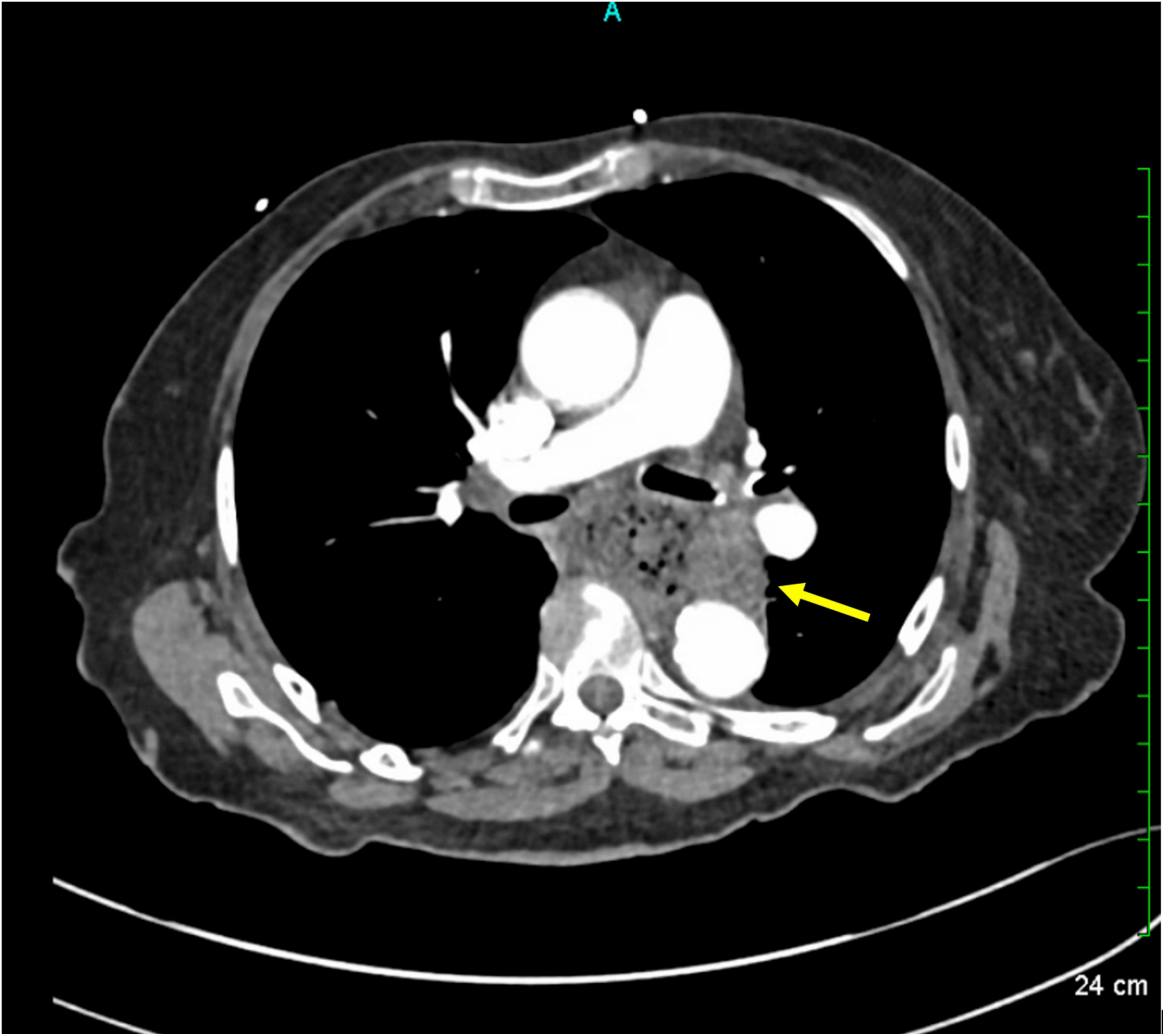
CT of the Chest With IV Contrast Demonstrating an Esophageal Mass Compressing the Trachea and Abutting the Left Atrium Yellow arrow: mass-like thickening involving the distal thoracic esophagus and gastroesophageal junction IV: intravenous
